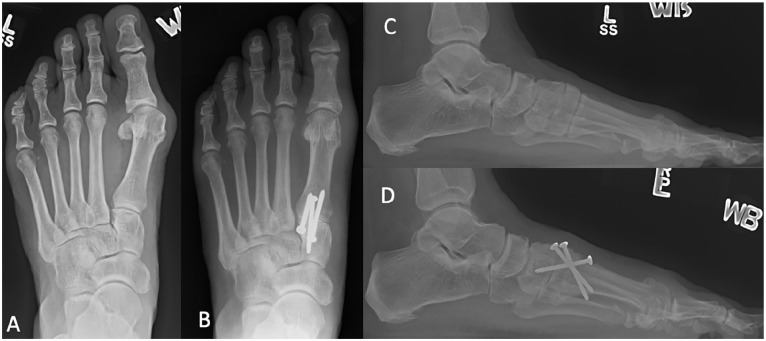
Left foot weightbearing radiographs of a patient undergoing open
